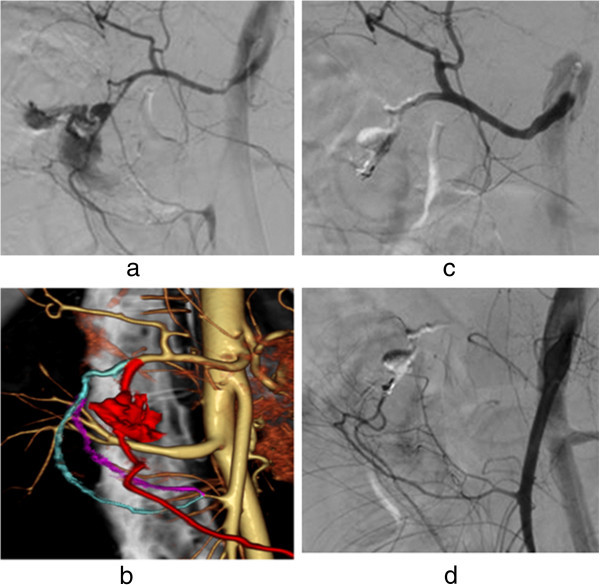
A case of bleeding from duodenal ulcer. (a) Celiac arteriography depicted the overlapping of the extravasation of contrast medium, gastroduodenal artery (GD), posterior pancreatico-duodenal artery (P-PDA) and anterior pancreatico-duodenal artery (A-PDA). (b) Hemorrhage-responsible arteriogram created by CT during abdominal aortography depicted that GD (red) was the responsible artery to hemorrhage but not P-PDA (purple) and A-PDA (blue). (c) Celiac arteriography following embolization of with n-butyl cyanoacrylate and microcoils depicted no extravasation of contrast medium. (d) Superior mesenteric arteriography depicted no extravasation of contrast medium.
